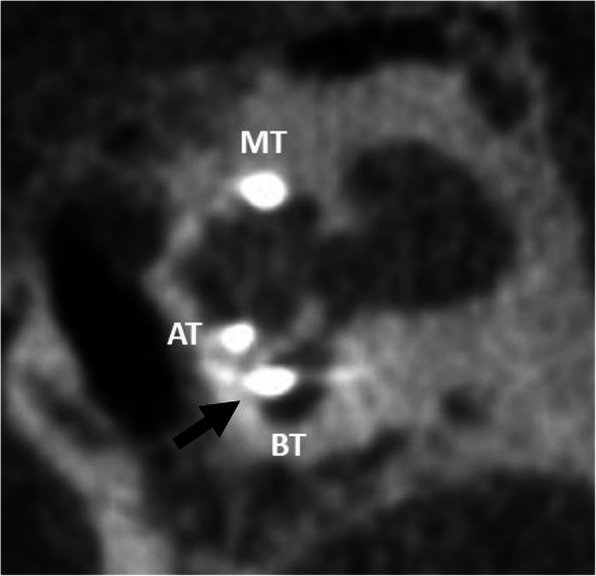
A 70-year-old female patient, with an electrode placed in the scala vestibuli. CBCT paracoronal image shows an electrode array located in the scala vestibuli (upper segment) of the cochlear duct (black arrow). BT – basal turn. MT – middle turn. AT – apical turn
